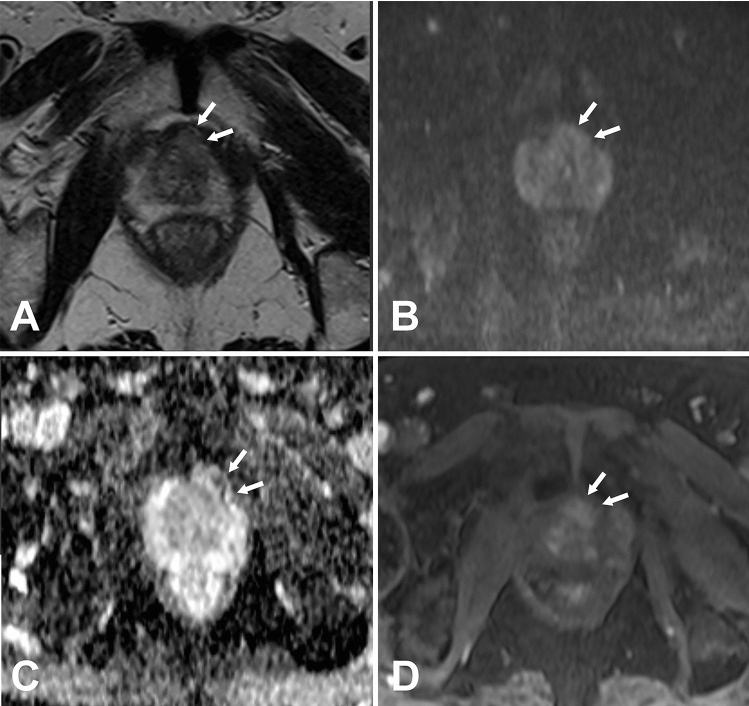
A 78-year-old man, the machine learning models misjudged 3 + 4 = 7 lesion (PI-RADS 4) in the transitional zone at the apex of the prostate (white arrow). The lesion showed slightly low signal on T2WI, no significantly high sign on DWI (*b* = 800), uneven signal reduction on ADC and positive dynamic contrast enhancement with early enhancement
